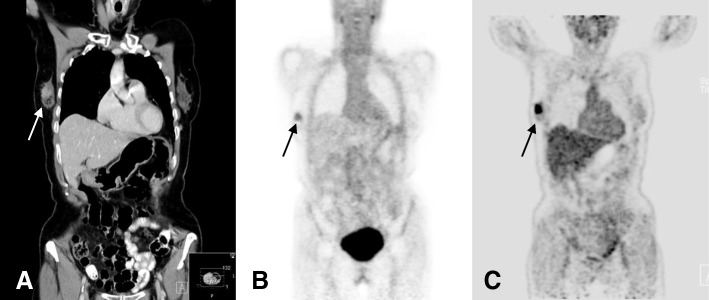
69-year-old patient with invasive ductal carcinoma (IDC) G2 with primary breast cancer prior to treatment. Coronal CT reconstruction shows contrast enhancement in a lesion with a diameter of 2.2 cm in the right breast (a). The tumor is visually detectable on ^68^Ga-Pentixafor PET with a corresponding SUVmax of 3.2 (b). On ^18^F-FDG PET/CT, the lesion demonstrates a significantly higher tracer uptake (SUVmax of 16.5) (c)
